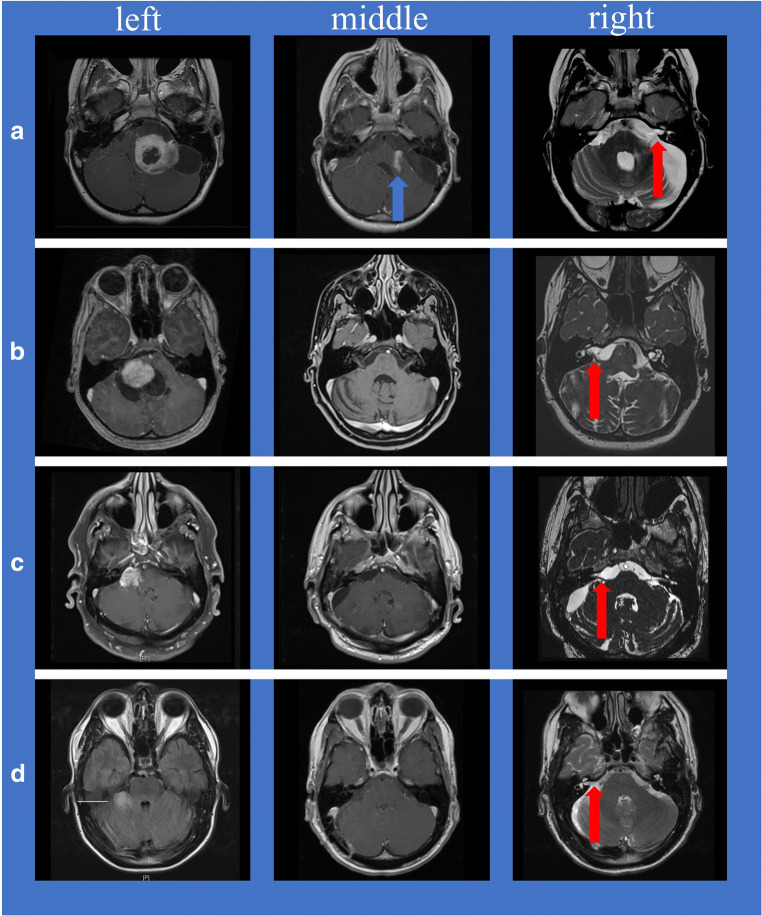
a Left (case 1), b left (case 2), c left (case 3), d left (case 4): Preoperative axial contrast-enhanced T1-weighted magnetic resonance imaging scans of each patient with PA-CPA (A+B: pediatric PA-CPA presented typically with a cystic cerebellar mass with enhancing mural nodule; C+D: adult PA-CPA presented atypically with only solid tumor components). a Middle, b middle, c middle, d middle: Postoperative axial contrast-enhanced T1-weighted magnetic resonance imaging scans; a gross total resection (GTR) of the PA-CPA was achieved in three cases (3/4). In one case, only a subtotal removal of the tumor was performed (blue block arrow). a Right, b right, c right, d right: Postoperative axial T2-weighted magnetic resonance images; anatomical facial nerve preservation was achieved during surgery in all cases (red block arrows)
